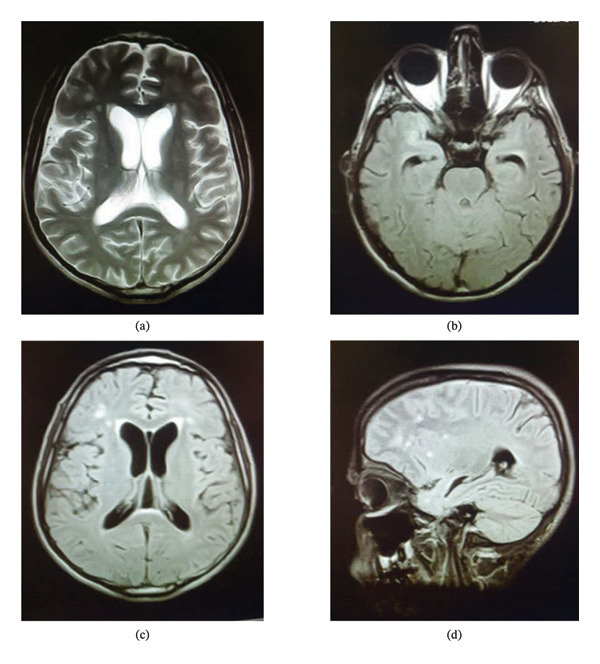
The brain MRI of the patient. (a) Hydrocephalus; axial T2W (TR/TE 5000/96) image without contrast, (b) hyperintensity lesion in the right temporal lobe; axial T1W (TR/TE 550/8.7) image without contrast, (c) demyelinating lesions in the right and left frontal lobes; axial T1W (TR/TE 9000/92) image without contrast, and (d) demyelinating lesions in the frontal lobe; sagittal T1W (TR/TE 9000/89) image without contrast.
